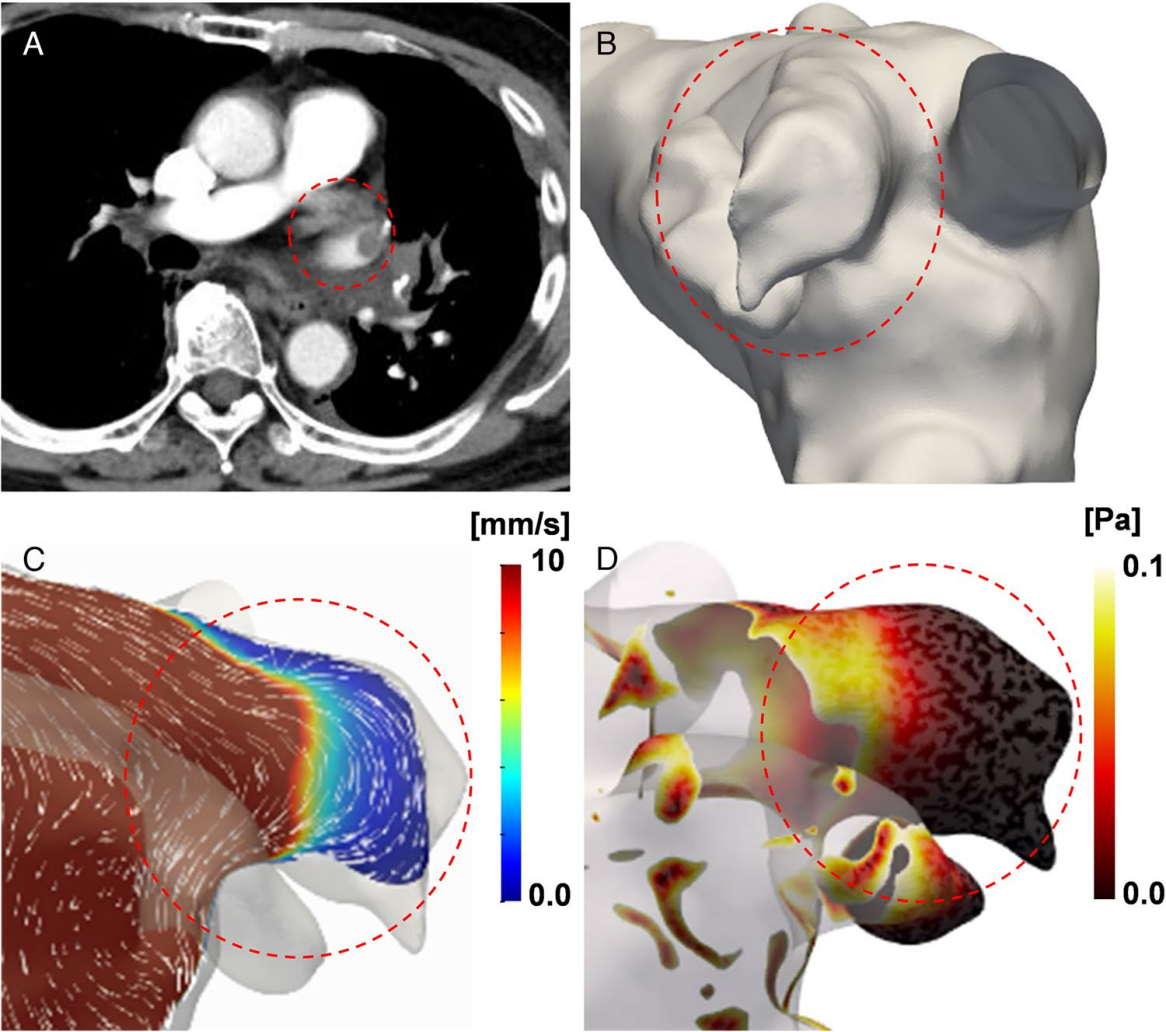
A Contrast-enhanced computed tomography was performed within 1 week after surgery. In this case, a thrombus was detected in the pulmonary vein stump (circle). B Image data in a Digital Imaging and Communications in Medicine format were transferred into three-dimensional patient-specific geometries, and the pulmonary vein stump was reconstructed (circle). C The computational fluid dynamics (CFD) method analysed the blood flow velocity vector. In this case, the low-velocity area presented in blue is shown in the pulmonary vein stump (circle). D The CFD method analysed the wall shear stress (WSS). In this case, low WSS area presented with dark red is shown in the pulmonary vein stump (circle)
